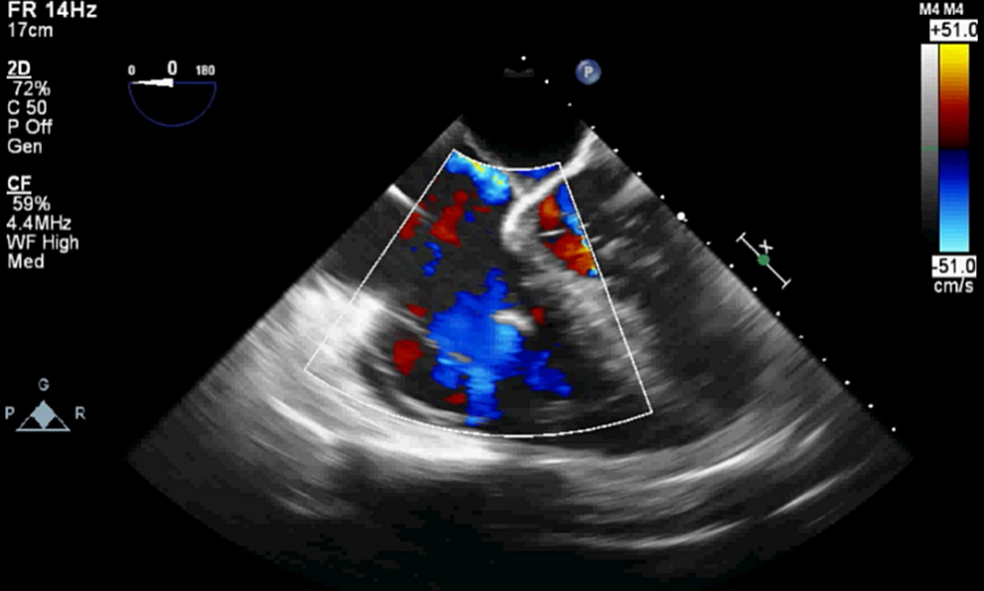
Transesophageal echocardiography (TEE) image demonstrating almost no tricuspid regurgitation (TR) on second presentation for liver transplantation after aggressive volume removal (mid-esophageal 4-chamber view).
